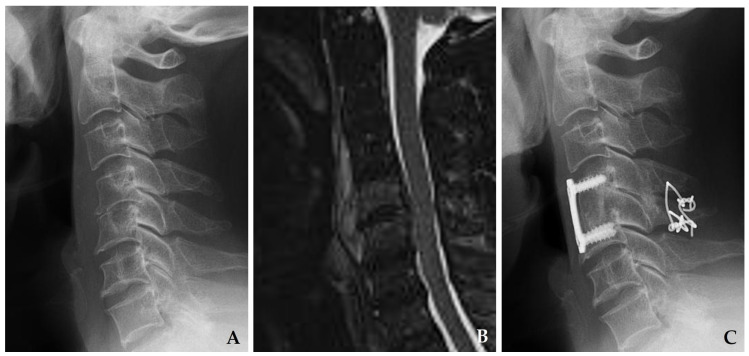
Initial plain radiograph (A) and magnetic resonance imaging (B) demonstrate disc space narrowing at C4–C5 with retropharyngeal and epidural abscesses. The patient underwent C4–C5 discectomy with autograft, anterior plating, and posterior wiring (C), achieving solid fusion and complete resolution of spondylodiscitis at one year postoperatively.
